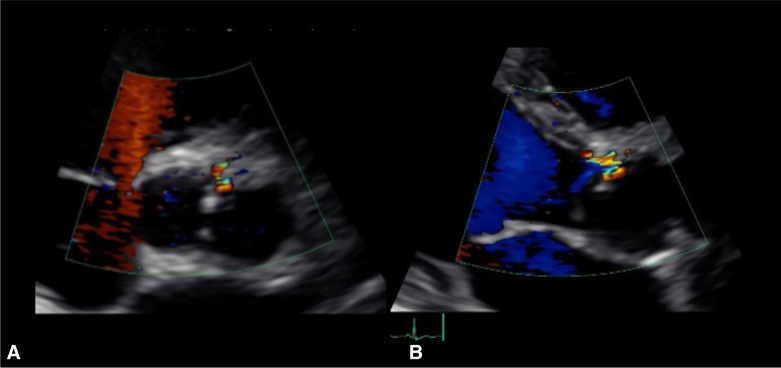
Latest transthoracic echocardiography at 6 years. Trace aortic regurgitation from the anterior commissure. A, Short-axis; B, long-axis.
